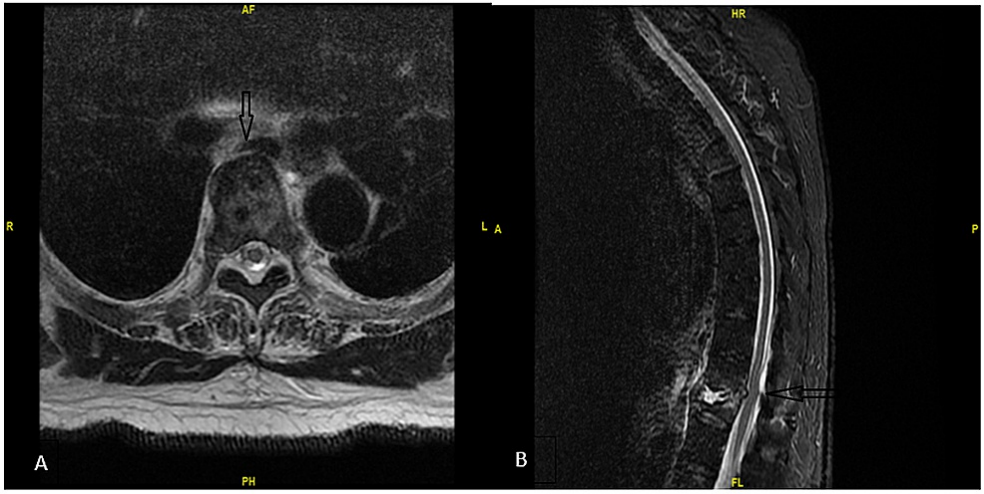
MRI of the thoracic and lumbar spine with and without contrast A: MRI axial view revealed discitis osteomyelitis involving the T11-T12 intervertebral disc space. B: the sagittal view showed there was a collapse of the T12 vertebral body, significant surrounding paravertebral soft tissue, and abnormal enhancing soft tissue was noted surrounding the thecal sac at the level of the T11-T12 vertebra. There may have been an area of significant compression of the thecal sac MRI: magnetic resonance imaging
